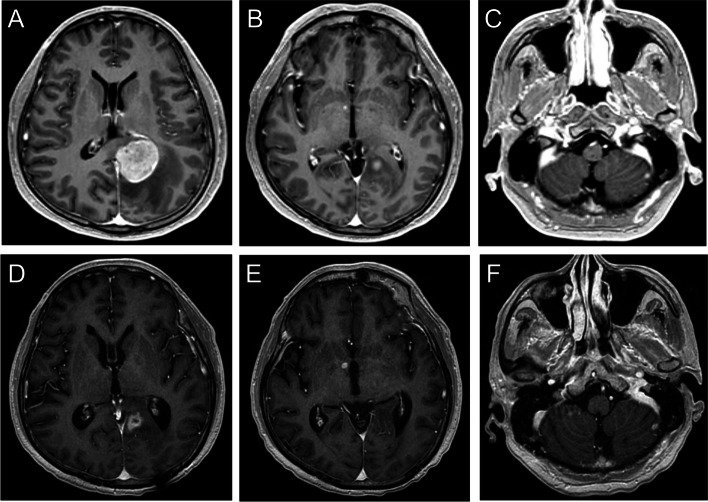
T1-weighted gadolinium-enhanced brain MRI of a 64-year-old man. Olioprogression lesions were noted in left occipital lobe (A), right basal ganglia (B), and left cerebellum (C). The patient continued afatinib after surgically removing the left occipital tumor with adjuvant WBRT. After a 6-month follow-up, the tumor was controlled in the left occipital (D) but progressed in the right basal ganglia (E) and left cerebellum (F)
